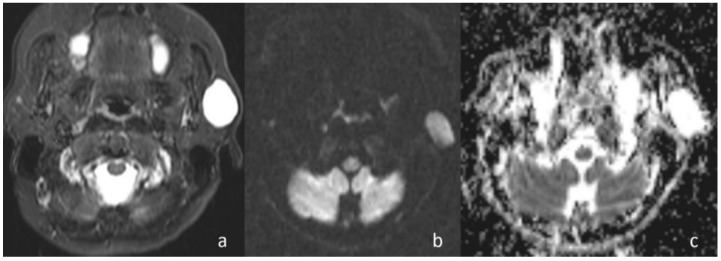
Pleomorphic adenoma of the parotid gland. (a) Axial T2-weighted image (T2WI) shows a mass in the left parotid gland; (b) the lesion shows low signal intensity on DWI; and (c) the mass is hyperintense on the ADC map (ADC value of 1.55 × 10^−3^ mm^2^/s).
